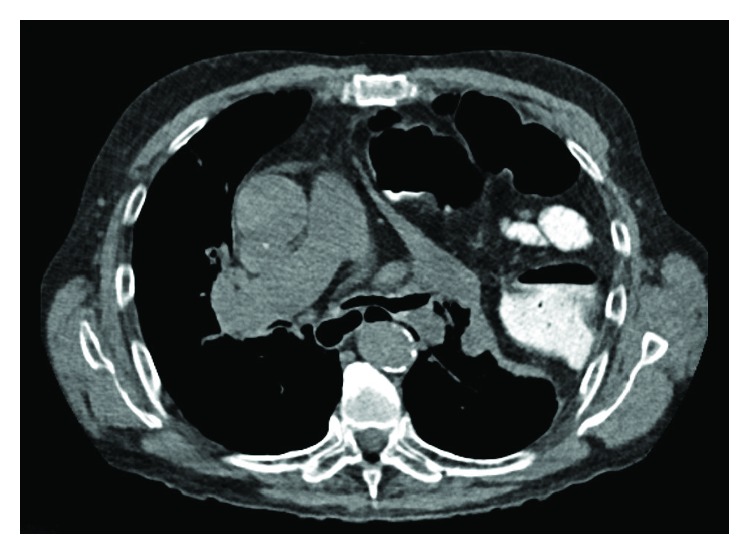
Axial CT scan demonstrating the diameter of the diaphragmatic hernia.
